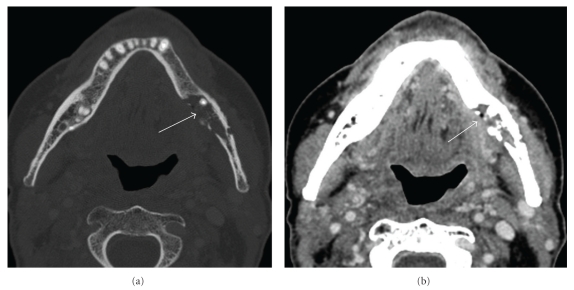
Mandibular osteoradionecrosis: (a) Axial contrast-enhanced CT of the mandible (bone window) shows destruction of the mandible, including along the lingual cortex and loss of the normal trabecular pattern (arrow). (b) Axial contrast-enhanced CT (soft tissue window) shows no evidence of an enhancing soft tissue mass. Linear enhancement (arrow) represents mucositis.
